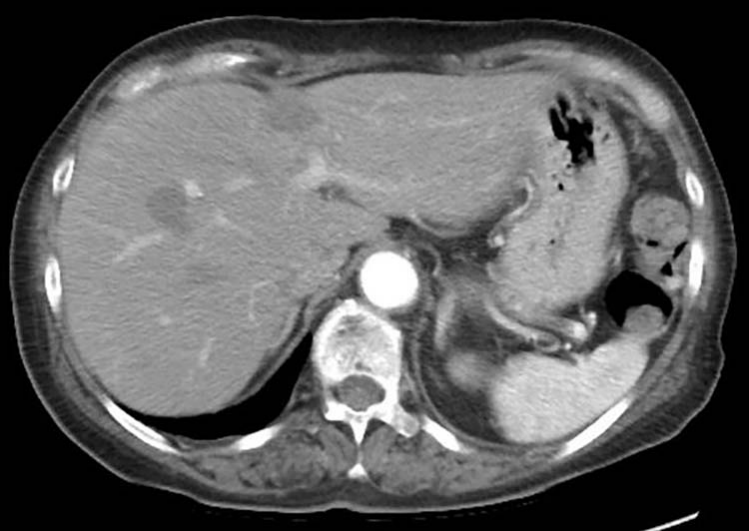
CT scan at 3 months postoperatively showed no recurrence.
